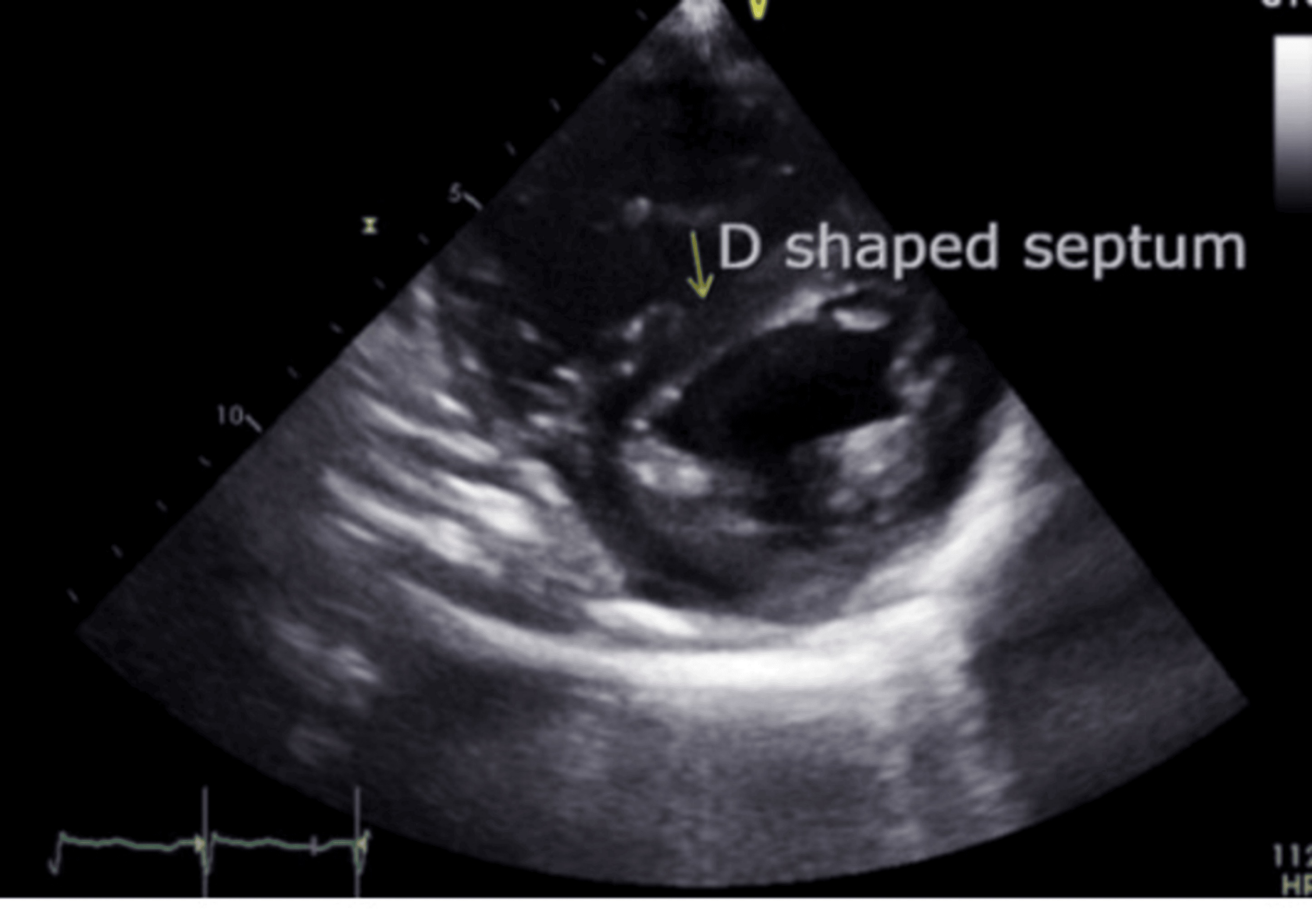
Echocardiogram shows the D-configuration, characteristic of right ventricular overload in the context of elevated pulmonary arterial pressure.
